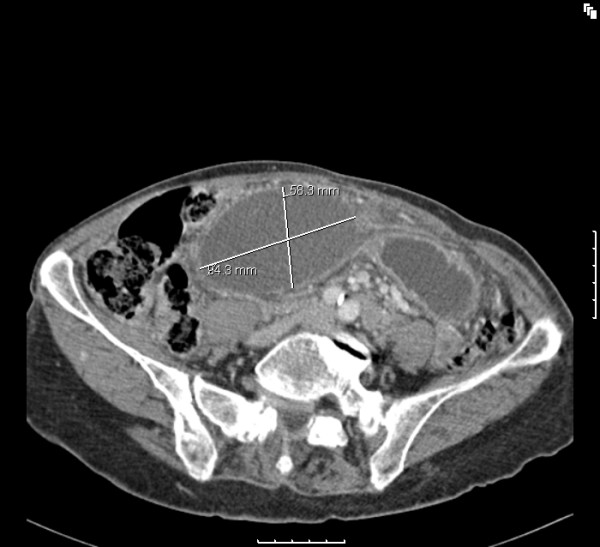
CT scan of the abdomen in horizontal section showing a cystic mass.
